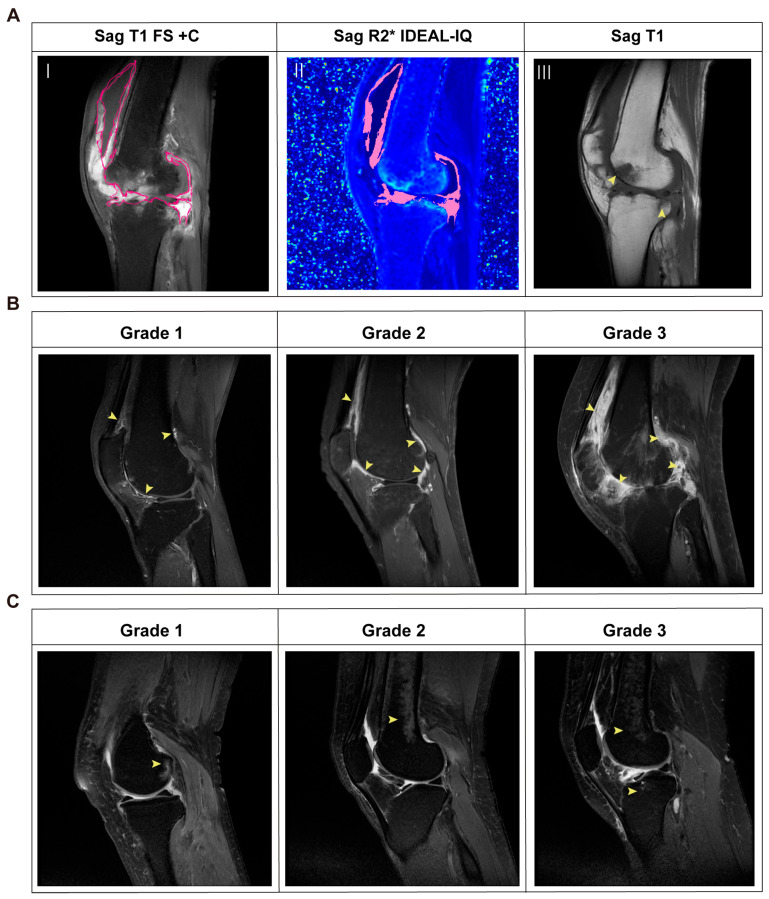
Assessment of synovial iron deposition and associated structural joint damage based on MRI. (A) MRI images from a patient used for evaluation of synovial iron and bone erosion. (I) Synovitis on sagittal T1 FS + C images (outlined in pink). (II) Iron examination in the synovium on sagittal R2* IDEAL-IQ images (outlined in pink). (III) Bone erosion (shown in yellow arrows) on sagittal T1 images. (B) Grading of synovitis on T1 FS + C images. (C) Grading of BME on T2-FS images. Grade 1 = mild, Grade 2 = moderate, Grade 3 =severe. Sag, sagittal; BME, bone marrow edema; T1 FS + C, T1-weighted fat suppression contrast-enhanced; IDEAL-IQ, Iterative Decomposition of Water and Fat with Echo Asymmetry and Least-Squares Estimation; T2-FS, T2-weighted fat suppression.
